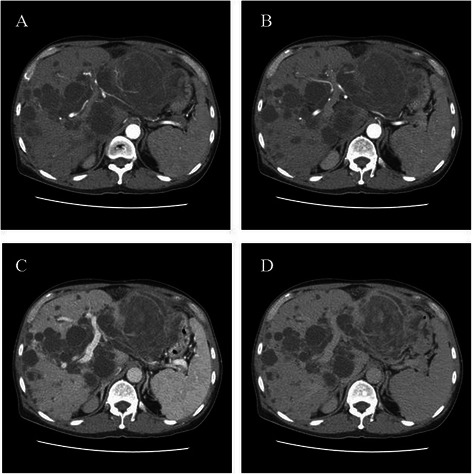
Contrast-enhanced triphasic liver CT of patient with intrahepatic biliary cystadenocarcinoma. The large (14 × 9.5 cm) cystic tumor occupying left lateral liver sector had diffuse mural soft tissue and fine tumor vessels. Faint arterial blushes with whirling appearance were also noted within the tumor and they faded throughout the dynamic study. Multiple liver cysts of various sizes were also found at the right liver lobe. a and b: arterial phase; c: portal venous phase; d: delayed venous phase
